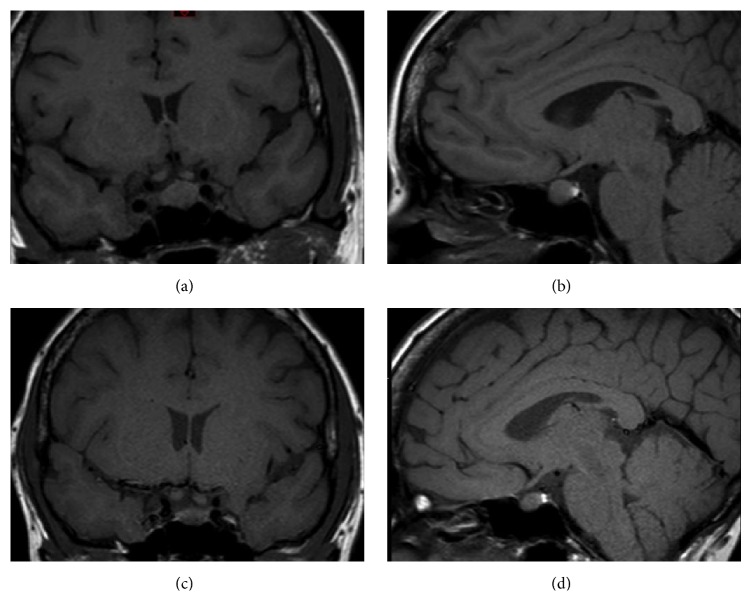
Sella MRI images showing pituitary enlargement at presentation (a, b) and 4 months after embolization (c, d), showing significant reduction in size.
